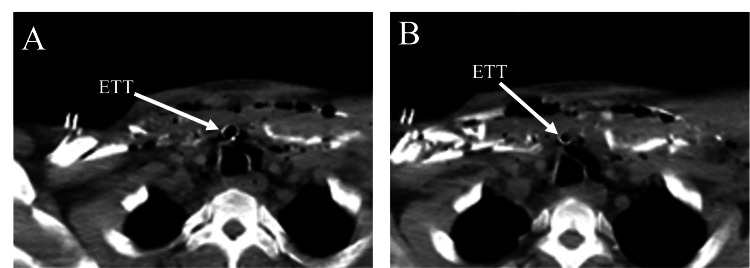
Axial CT chest showing perforation of endotracheal tube (ETT) through the tracheal injury
